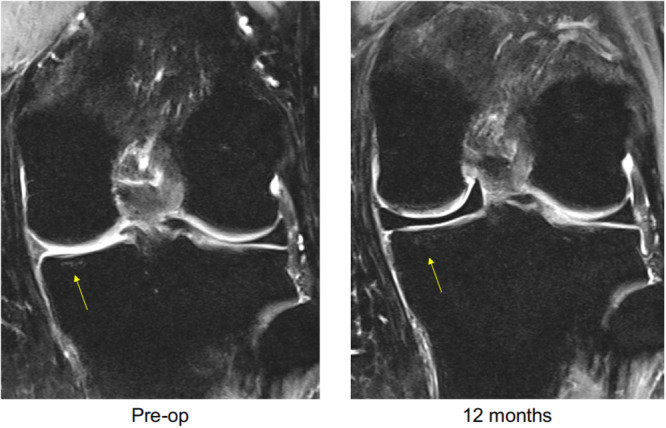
An example of a 51‐year‐old male who was diagnosed pre‐operatively as having a subchondral bone marrow lesion (left). Magnetic resonance imaging findings 12‐month post‐treatment with non‐anchored, interpositional knee implant (right).
